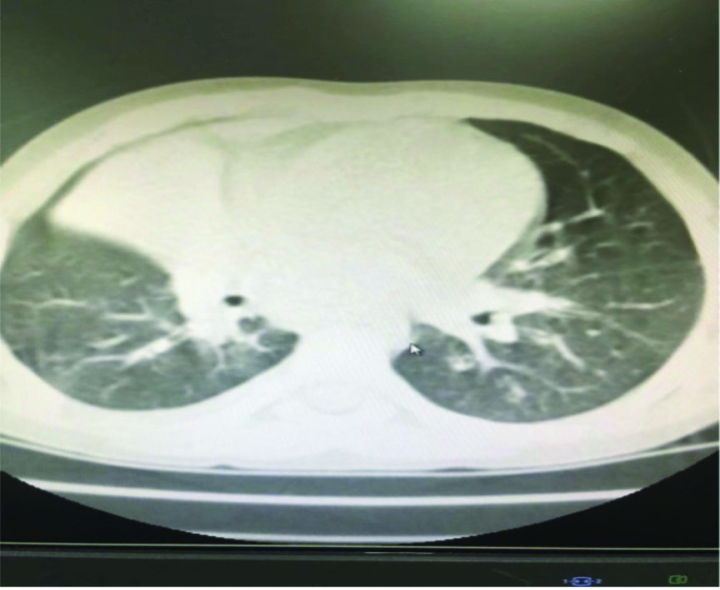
Computed tomography (CT) scan showing right middle lobar consolidation.
